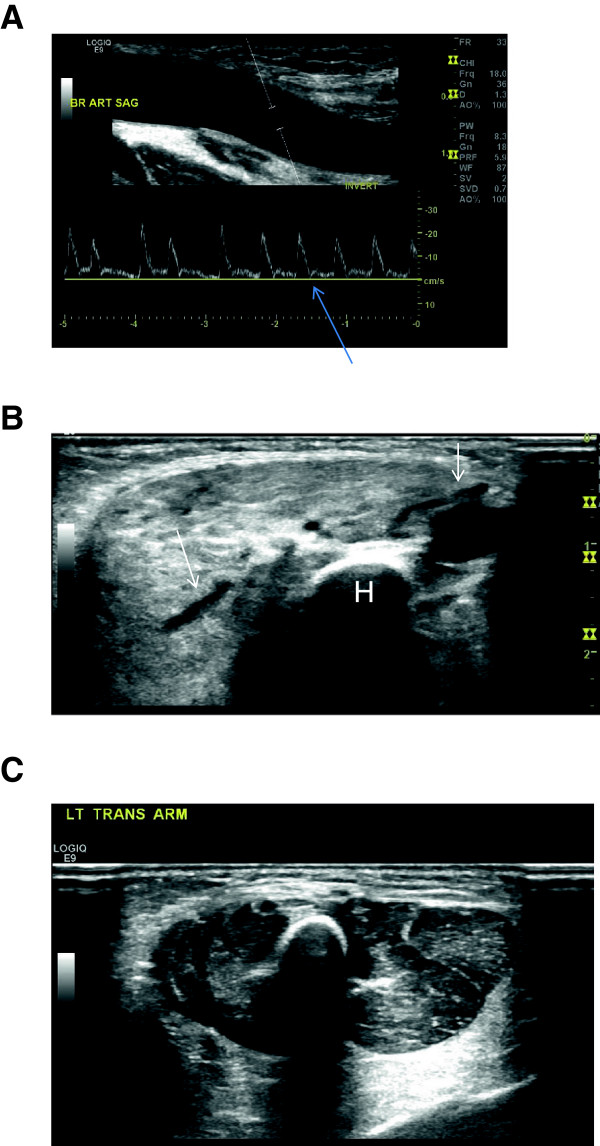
Ultrasound: 2A) Doppler waveform obtained from the right brachial artery reveals absence of an expected transient reversal of flow (arrow). Also seen is massive dilation of the brachial artery (calipers in artery). 2B) Dilated vessels (arrows) are seen coursing through the abnormally hyperechoic, poorly defined and enlarged musculature. Humerus (H). 2C) Normal left arm for comparison
